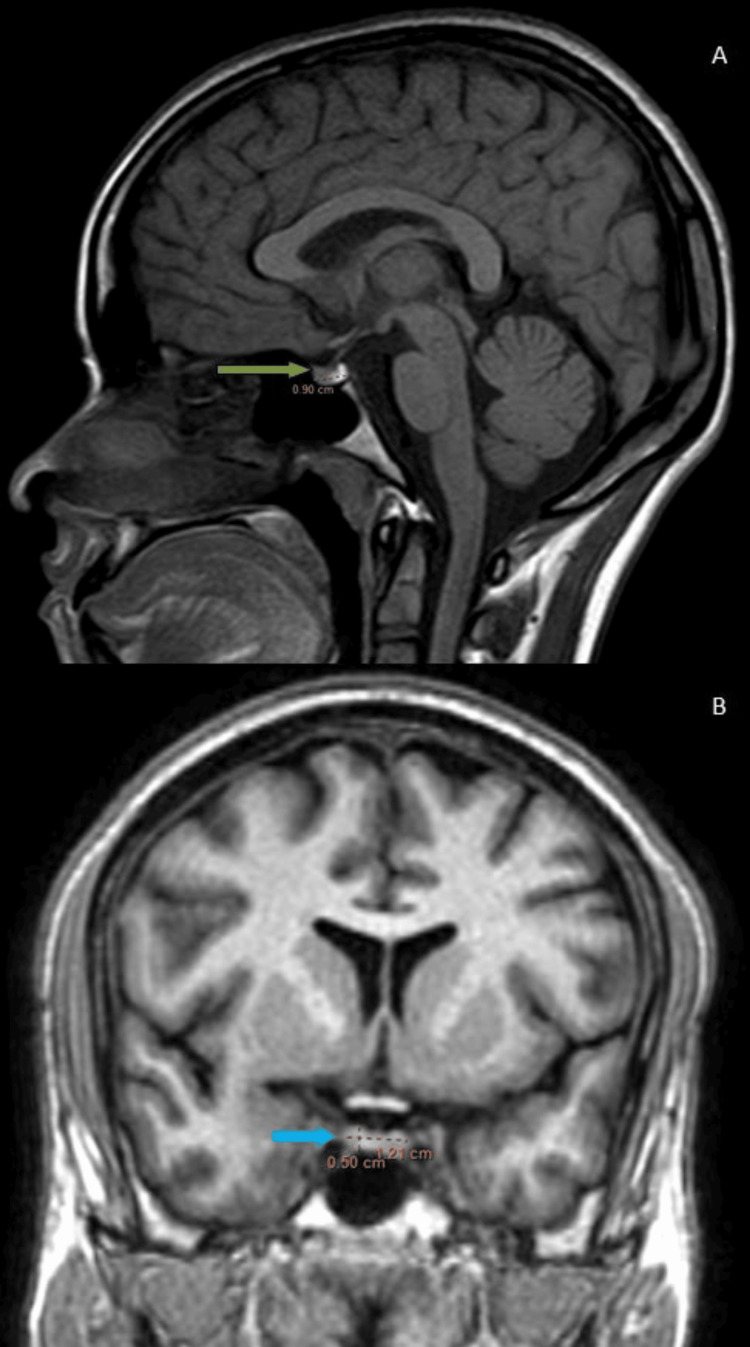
(A, B): MRI of the sella turcica. (A) T1 mid-sagittal: the pituitary gland in a 65-year-old female shows an anteroposterior of 9 mm (green arrow). (B) FLAIR mid-coronal: the pituitary gland of the same female shows a transverse dimension and height of 12 mm and 4 mm, respectively (blue arrow). The pituitary gland volume is 216 mm3
